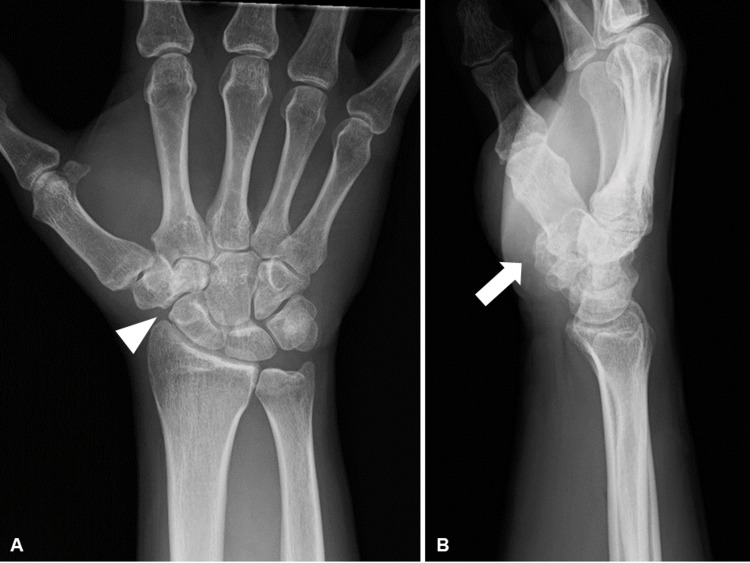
Plain radiography at one year postoperatively. (A) Anteroposterior and (B) lateral views of the right wrist (arrowhead shows joint space of the scaphotrapeziotrapezoid joint, and arrow shows volar fragment of the trapezium).
